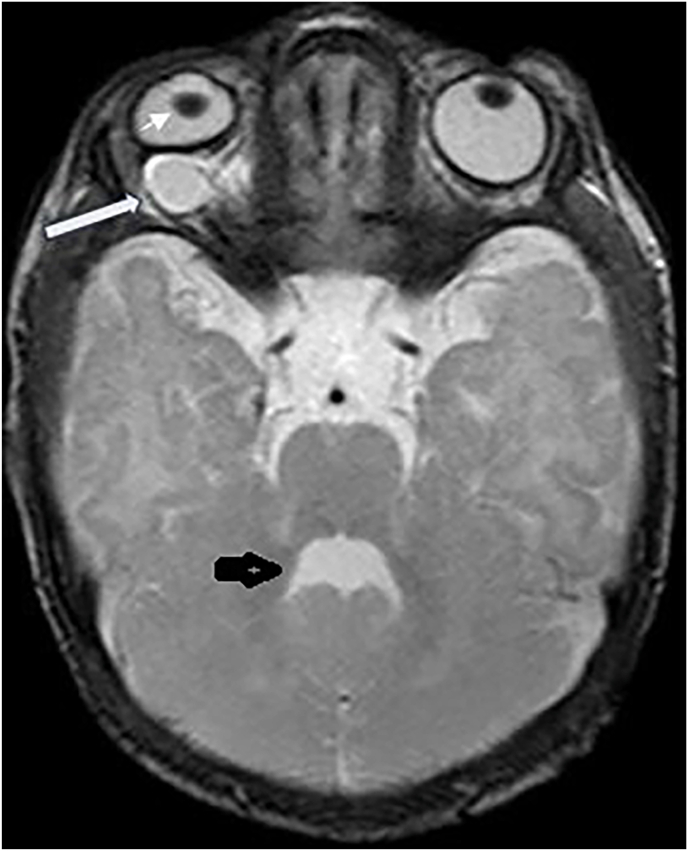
Axial T2 weighted MR image shows right retrobulbar cysts (long white arrow) with deformity of the globe and right microphthalmia and lens subluxation. Fourth ventricle shows “batwing or umbrella sign” characteristic of Joubert syndrome (short black arrow).
